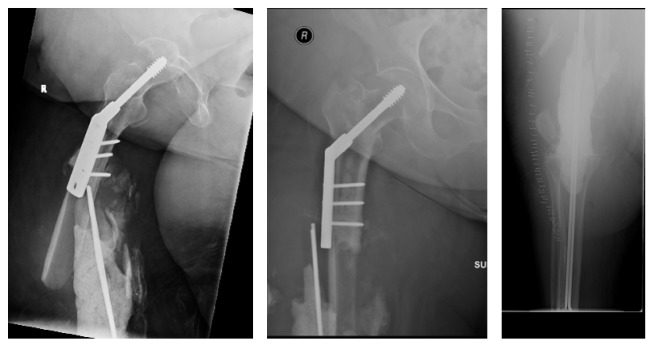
AP and lateral radiographs of right hip and lateral radiograph of right knee at presentation. Radiographs with antibiotic spacer over intramedullary K wire in tibia and extramedullary in femur. DHS in proximal femur.
